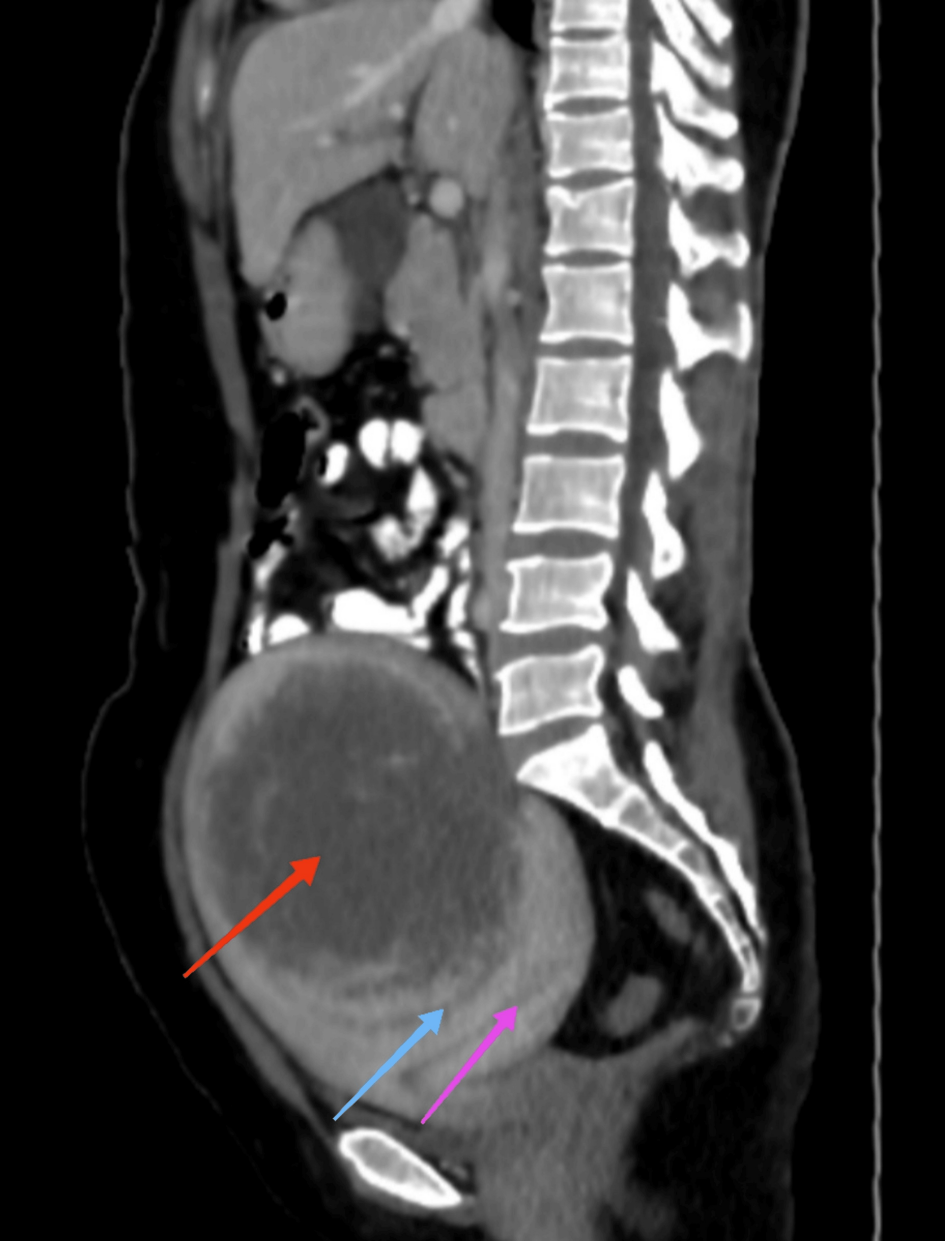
Computed tomography scan in sagittal section showing huge fundal fibroid arising from the anterior wall of the uterus The red arrow shows the mass, the blue arrow shows the capsule of the fibroid, and the pink arrow shows the endometrial cavity
